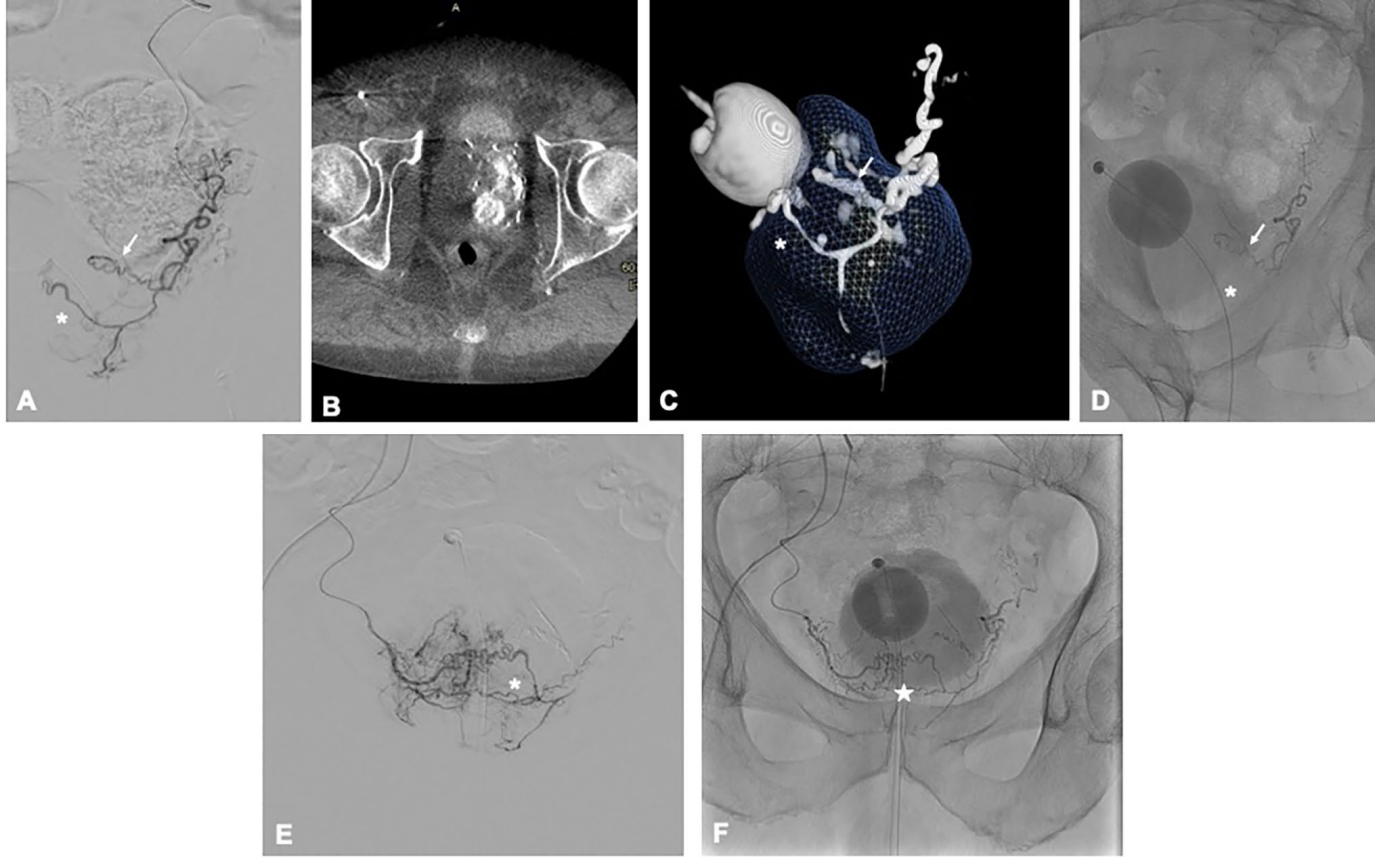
PAE with NBCA in an 80-year-old patient with an IPSS of 23. A Left oblique angiographic image shows the left prostatic artery dividing into a posterior–cranial branch directed to the third lobe (arrow) and an anterior–caudal branch (asterisk). B Axial Cone-Beam CT image acquired after contrast injection (1 mL/s flow rate, 4 mL total volume, 6-s acquisition delay) demonstrates opacification of the entire left prostatic hemigland. C 3D reconstruction of the prostate (volume: 74 mL) shows the intraparenchymal branching (arrow and asterisk) of the left prostatic artery. D Post-embolization angiogram shows glue–oil cast in the posterior branch (arrow) but not in the anterior–caudal branch (asterisk). E Angiogram of the right prostatic artery reveals perfusion of the right hemigland and the untreated portion of the left hemigland (asterisk). F Final angiographic image demonstrates distribution of the glue–oil cast within the parenchymal branches of the prostate (star) and along both right and left prostatic arteries
